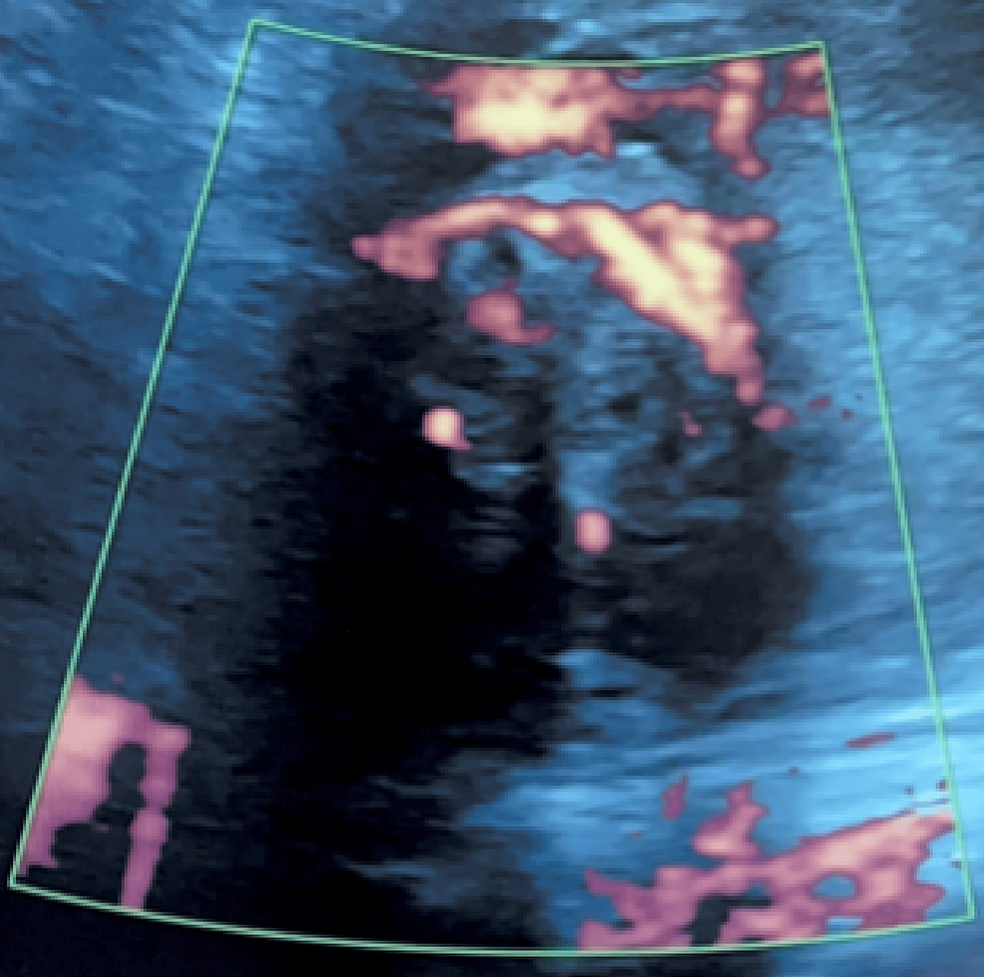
Ultrasound images of the uterus (transverse view) displaying a central cystic area with circumferential blood flow with no yolk sac or fetal pole.
